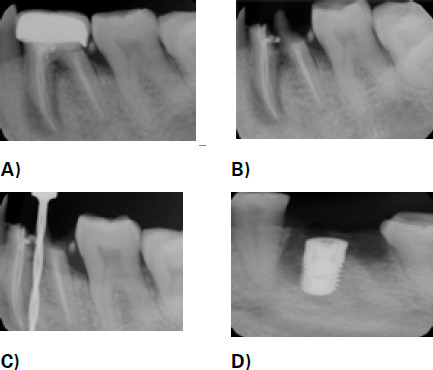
Radiographic image showing A) artificial crown with periapical radiolucency extending till furcation area and fracture of distal root at the level of the crest, B) sectioning of crown horizontally and separation in between mesial and distal roots, C) After osteotomy, checking for center point in between mesial and distal roots, D) Implant placement in the desired area after removing roots.
